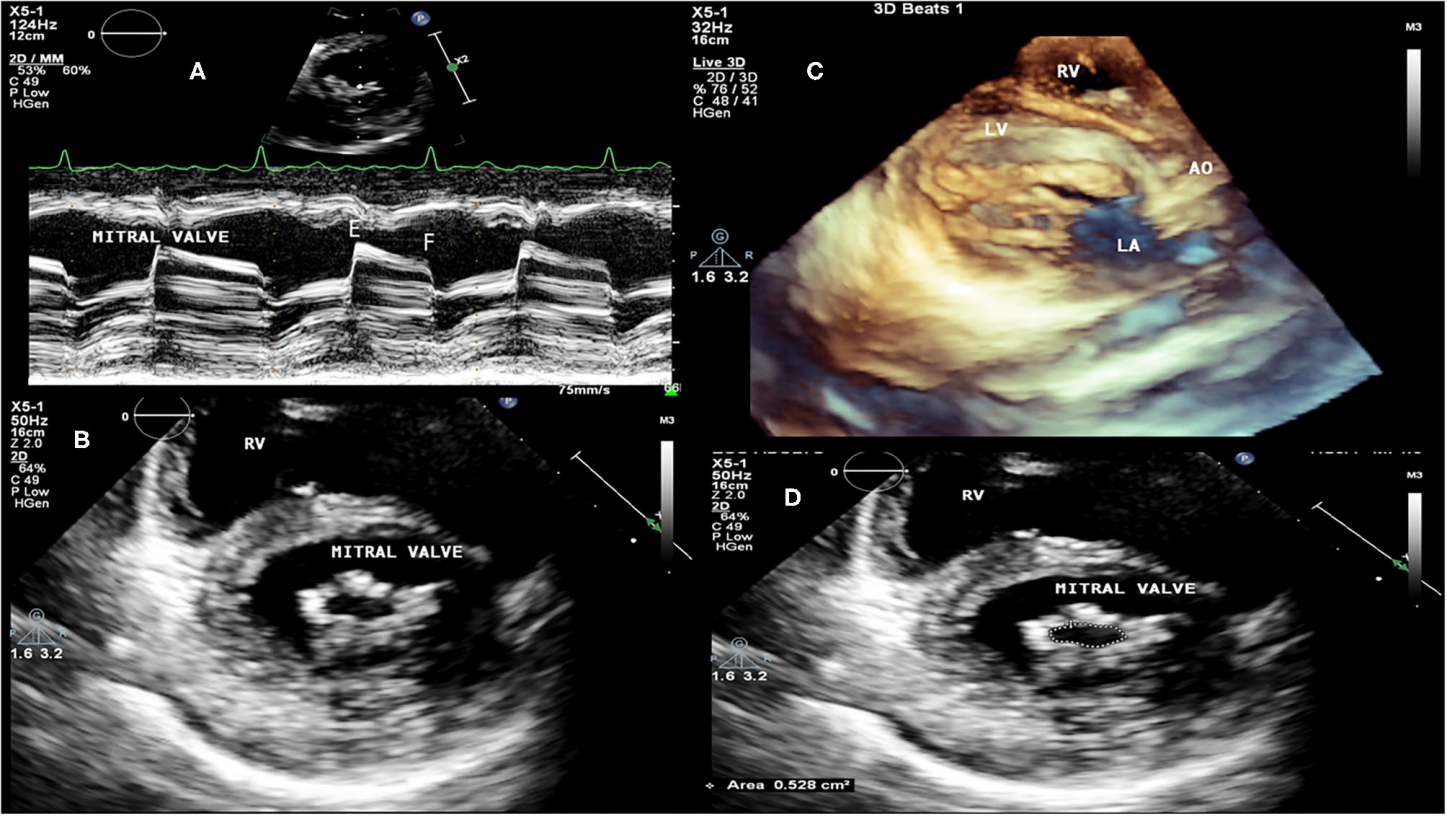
RHD (mitral valve stenosis). (A) M mode transthoracic echocardiography. Demonstration of patient with severe mitral valve stenosis (rectification of E to F slope), presenting atrial fibrillation. (B) 2D transthoracic echocardiography, transversal view, thickening of mitral valvular leaflets, “buttonhole” shape of the mitral valve. (C) 3D transthoracic echocardiography, mitral valve chordae fusion. (D) 2D transthoracic echocardiography, transversal view, measurement of the mitral valve area by planimetry: 0.528 cm^2^. RV, right ventricle; LV, left ventricle; AO, aorta; LA, left atrium.
